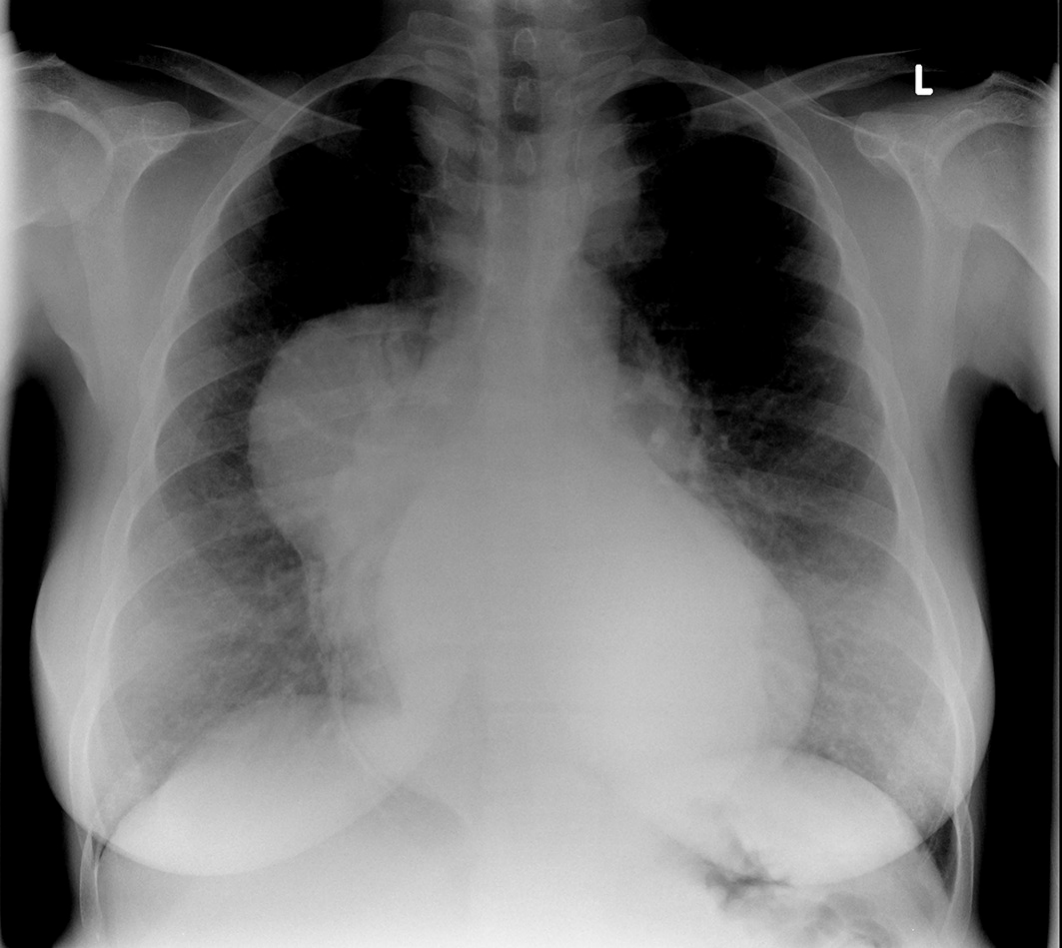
Review of a previous radiograph revealed paravertebral masses.
